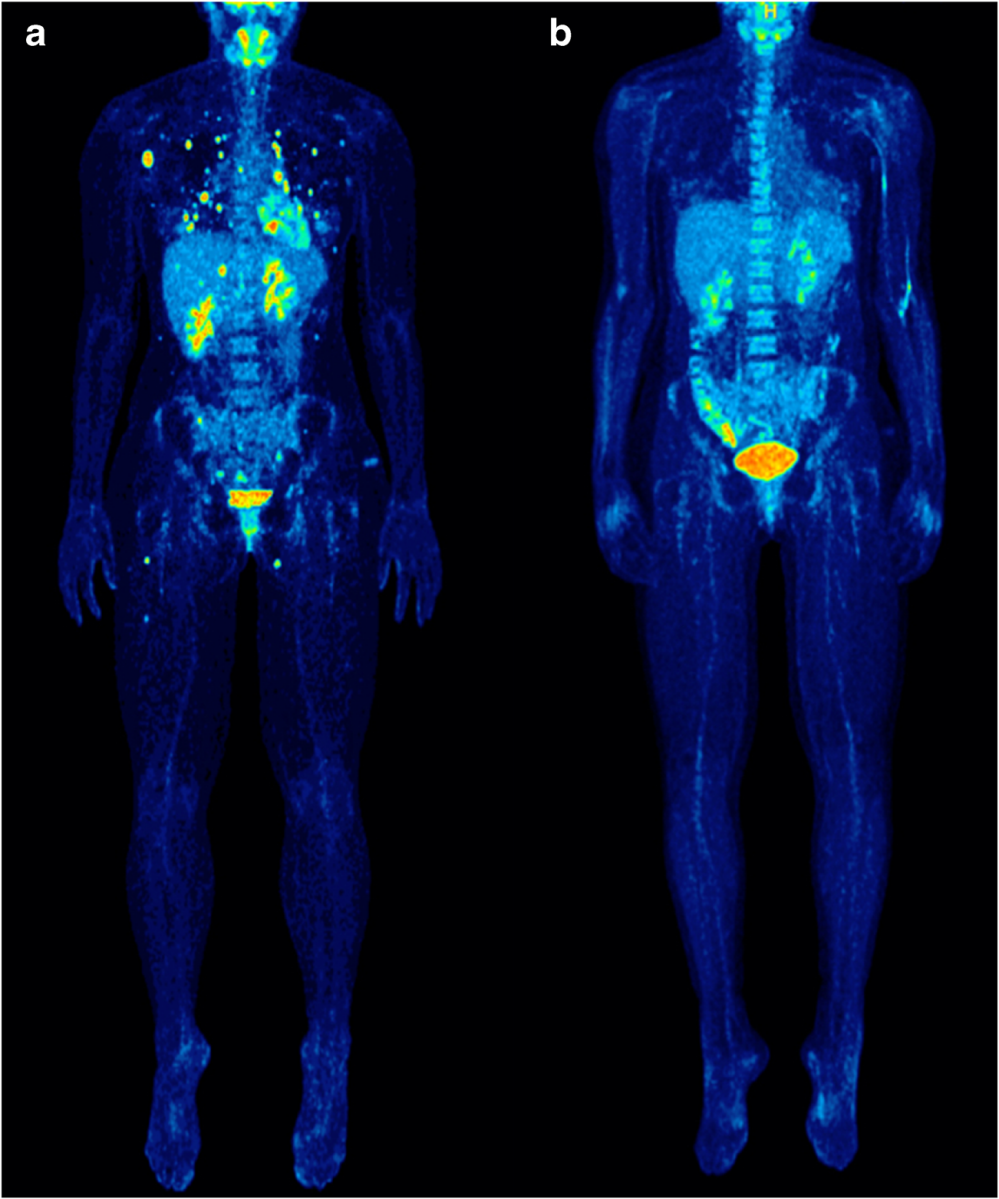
Maximum intensity projection (MIP) [^18^F]FDG PET/CT images of a 34-year-old woman with metastatic melanoma before initiation of immunotherapy with nivolumab/ipilimumab (A) and after administration of two cycles of treatment (B). Baseline PET/CT image shows multiple lymph node, pulmonary, hepatic, adrenal, soft tissue, and osseous metastases (A). Interim PET/CT shows a complete metabolic remission (CMR) of all baseline lesions. The patient demonstrated metabolic benefit (MB) according to both the EORTC and PERCIMT criteria (B). Moreover, diffusely increased [^18^F]FDG uptake is observed in the ascending colon and the bone marrow on interim PET/CT. At the time of writing, the patient was still progression-free having reached a PFS of 17.9 months
